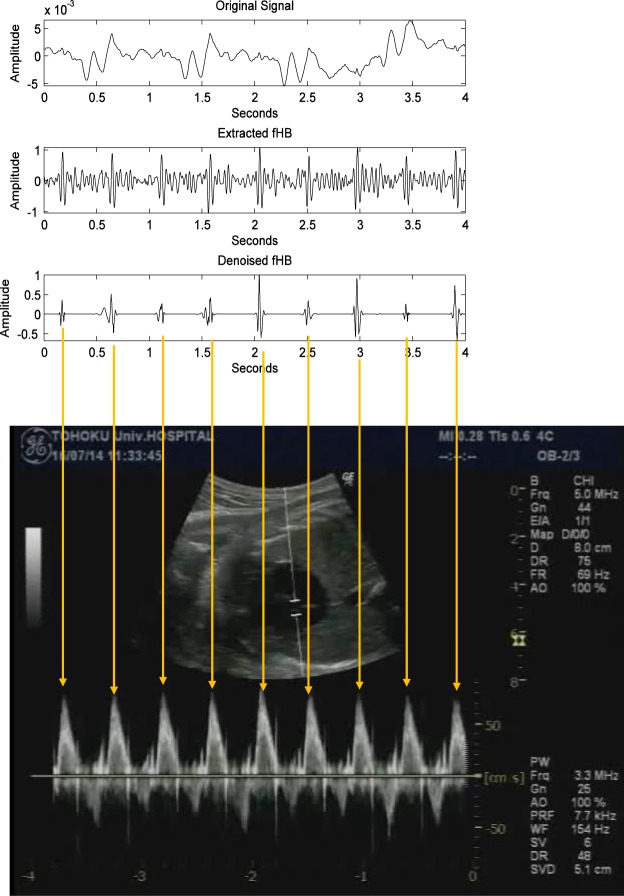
Beat by beat validation with pulsed Doppler M-mode images.
